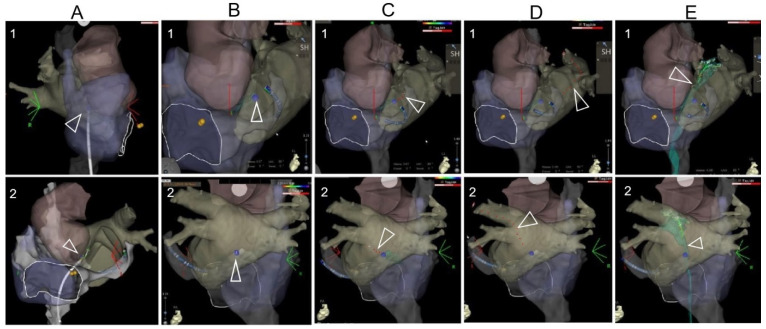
The TSP images under the visualization of a 3D mapping system. (A): After the 3D mapping of the right-sided anatomy, the CT anatomy was superimposed. The RF catheter was tracked during its moving from the superior part of the mapping anatomy. (B): The location of the TSP was identified and annotated on the 3D mapping anatomy. (C): The TSP needle was visualized and positioned on the annotated TSP point through the steerable sheath. (D): After visualization of needle puncture through the septum, it was replaced by the guidewire. The guidewire was tracked during its advancement to the PV on the 3D reconstructed anatomy. Note that 1 and 2 are modified projections for better visualization of the superimposed reconstructed anatomy (usually modified RAO and modified LL or PA projections). (E): After the TSP, the mapping catheter was introduced to the left chamber via the long sheath, and a FAM (fast anatomical mapping) of TSP was performed, shown as green color.
